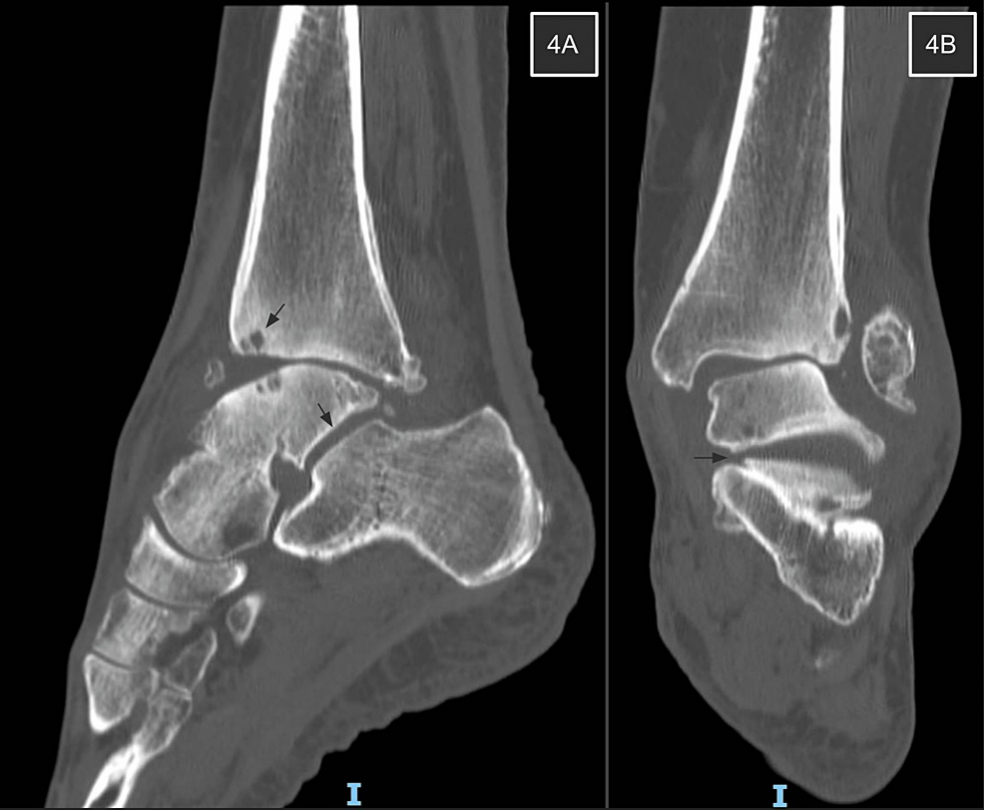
Ankle CT scan 16 months post-FVFG CT scans as shown in 4A and 4B reveal small periarticular cysts of the ankle joint and confirm the viability of the talus. Subtalar joint congruency is well preserved.
